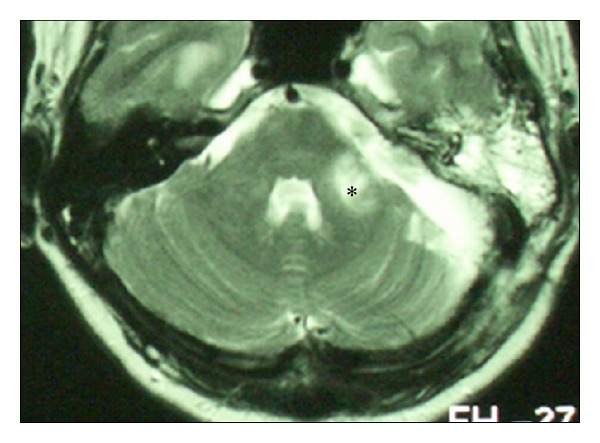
MRI of patient with peduncular venous infarction (asterisk) due to superior petrous vein injury.
